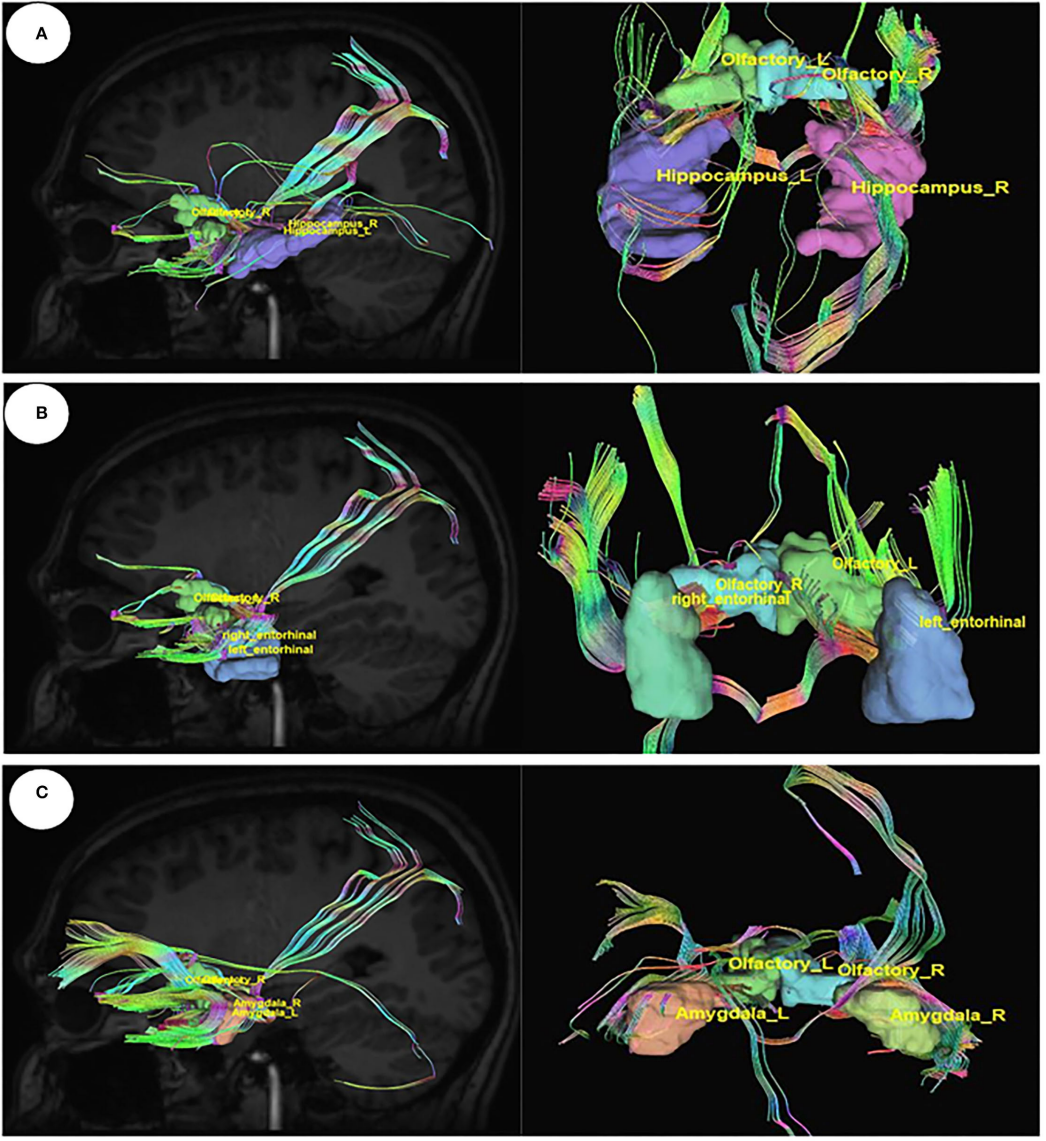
Diffusion MRI fiber tracking connecting the olfactory nerve with (A) the hippocampus, (B) the entorhinal cortex, and (C) the amygdale.
